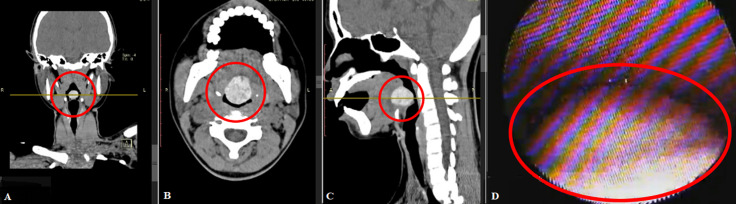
A, B, C) head and neck CT-scan; D) Fiber optic laryngoscope (FOL *)*; there is a soft tissue mass at the base of the tongue (red circle)
